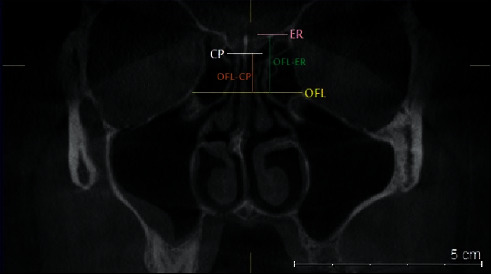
Coronal plan of CBCT demonstrating landmarks of TMS classification: orbital floor (OFL): yellow line, cribriform plate (CP): white line, ethmoid roof (ER): pink line, OFL-CP: orange line, OFL-ER: green line.
